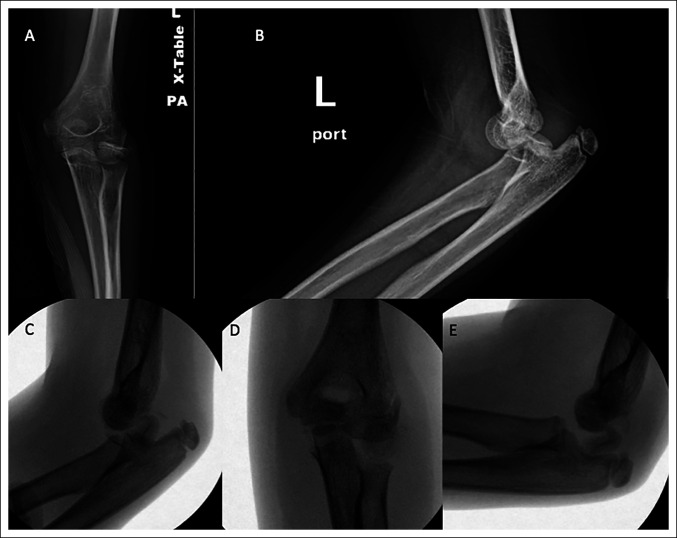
Images presenting left elbow injury radiographs. Radiographs showing anteroposterior (A) and lateral (B) views of the left elbow depicting a posterior elbow dislocation and a Salter-Harris II radial neck fracture.
